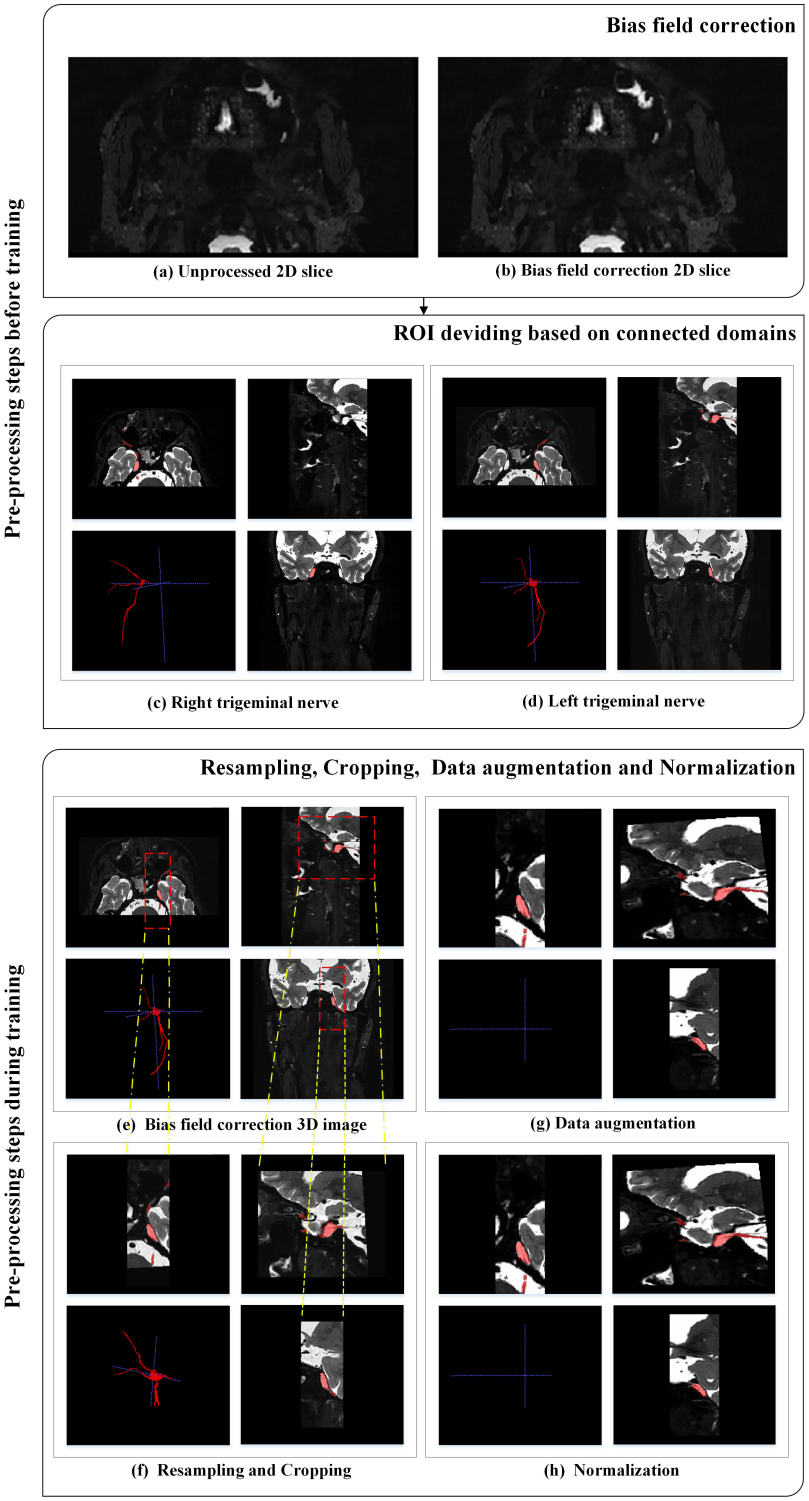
Pre-processing steps applied to each MRI case.
